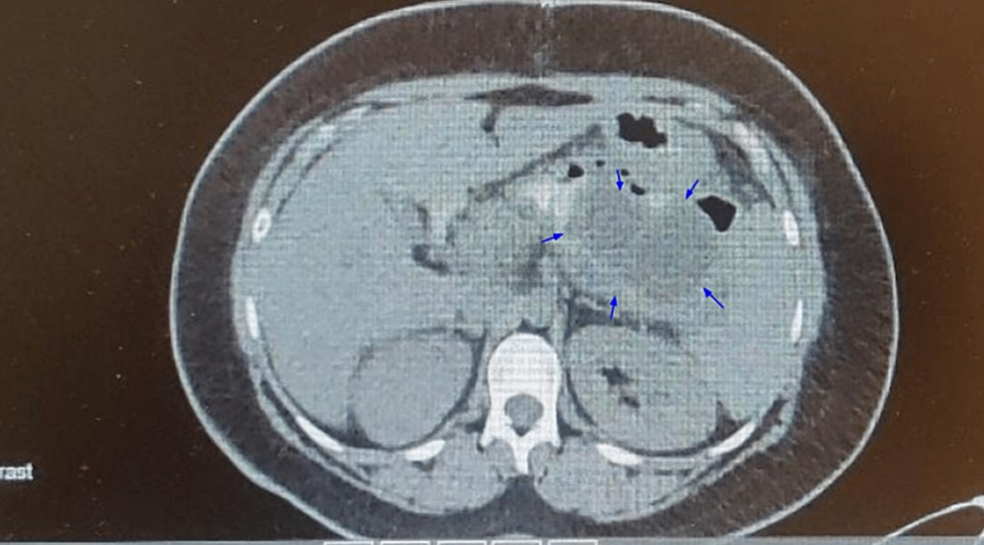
Oral contrast CT scan of the abdomen on postoperative day 8. Blue arrows point toward the pancreatic cyst, which is markedly reduced in size.
